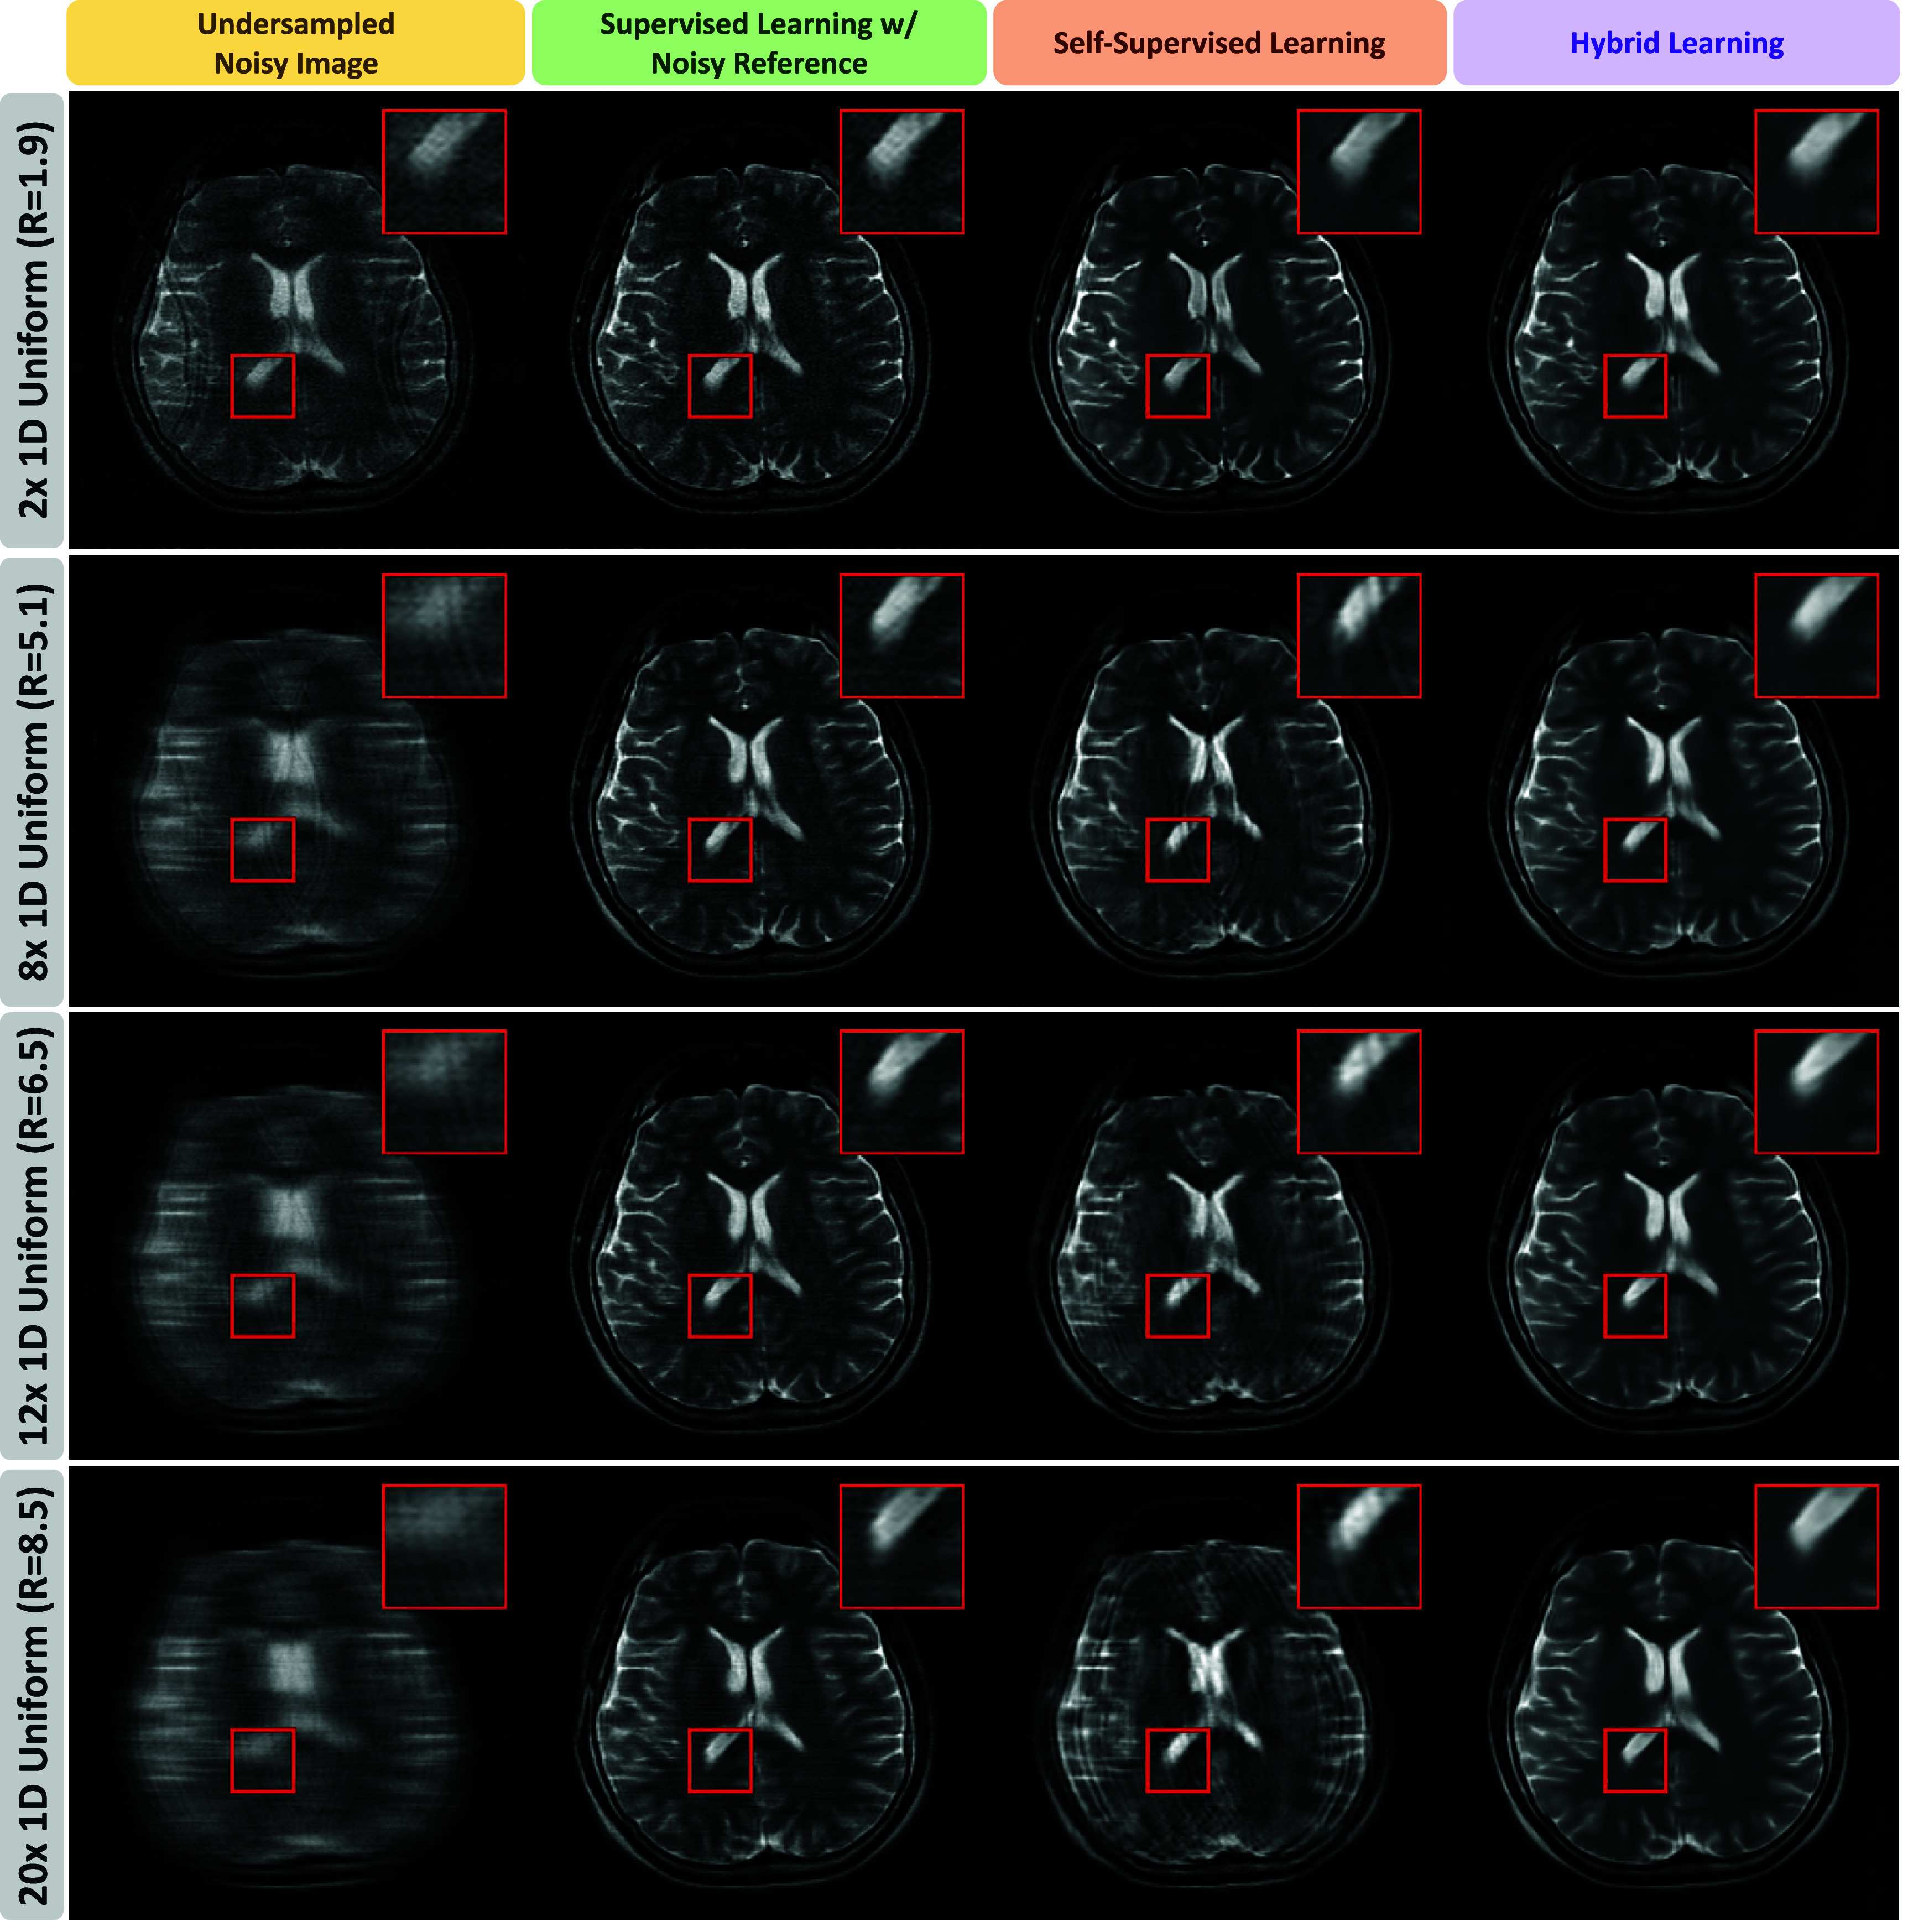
Brain MRI (0.3 T), Cartesian sampling at 2-fold, 8-fold, 12-fold, and 20-fold 1D undersampling. The corresponding case from figure 6 at other acceleration rates (2×, 8×, 12×, 20× 1D uniform), comparing hybrid learning with supervised and self-supervised learning.
